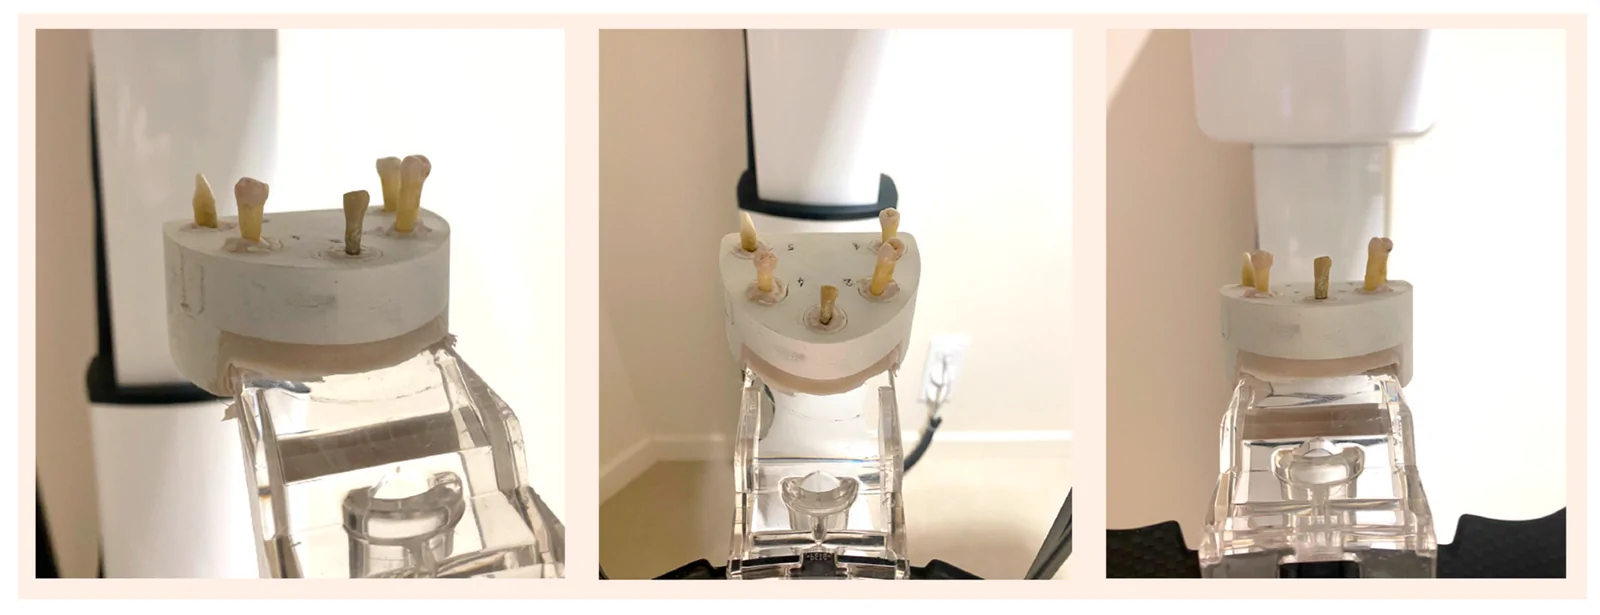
Three-dimensional-printed holder designed for reuse adapted to the CBCT headrest using high-performance alginate.
